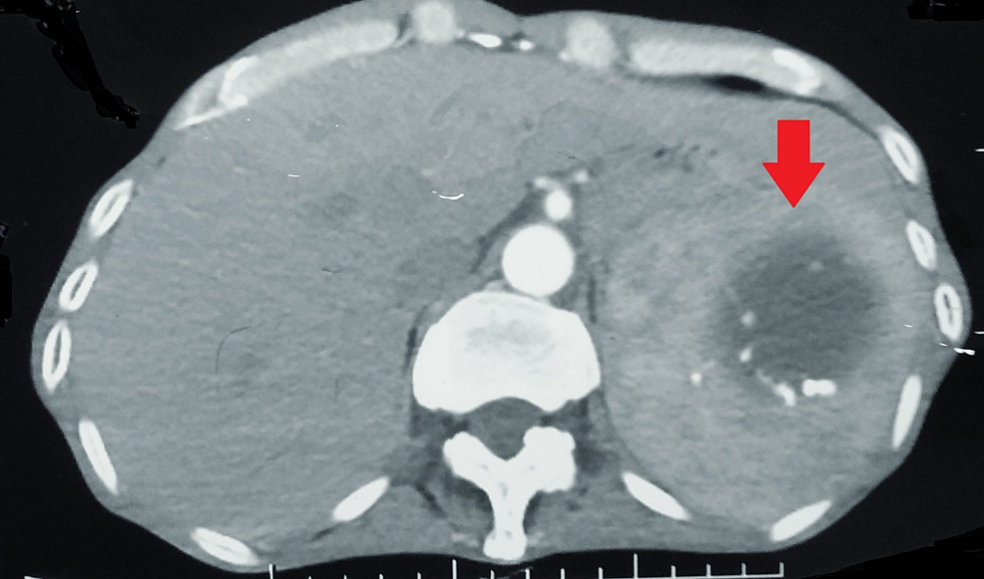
Computed tomography of the lungs showing a well-defined fluid-attenuating cystic lesion with peripheral wall calcifications in the spleen
